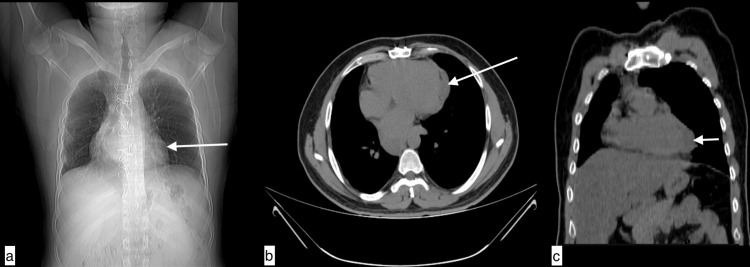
Pericardial hydatid cyst A 32-year-old male with a pericardial hydatid cyst presented with fever, dry cough, chest pain, and severe exertional dyspnea for two weeks. Topogram image (a), axial image (b), and coronal image (c) of non-contrast CT chest revealed a well-defined hypodense (+30 to +36HU) lesion in the right para oesophagal region extending into the middle mediastinum and along the pericardium with loss of fat planes with underlying myocardium and adjacent major vessels.
